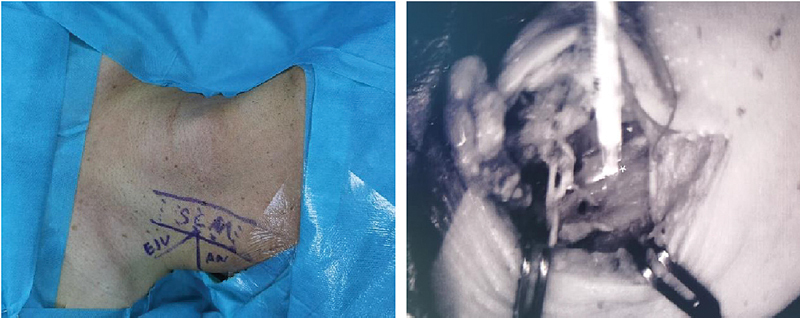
Using ultrasound mapping, we preoperatively marked the sternocleidomastoid (SCM) muscle, external jugular vein (EJV), accessory nerve (AN), and lymph nodes (left side). Indocyanine green (ICG) was injected cranially along the carotid sheath (white star) to trace the lymphatic channels and confirm their patency following lymphovenous bypass.
